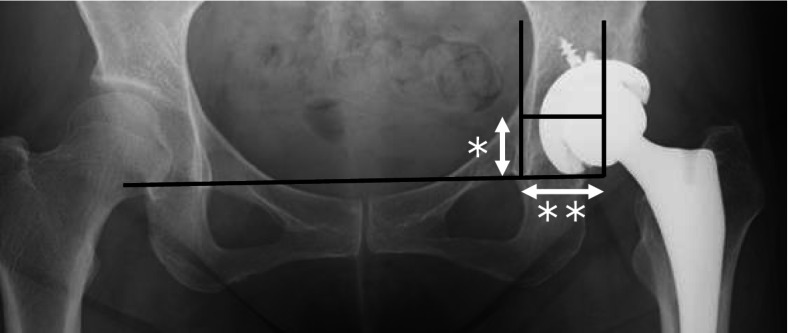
Postoperative anteroposterior X-ray radiogram of THA. The vertical distance (*) is defined as the distance from the lower edge of the bilateral the tear drops to the center of the hip joint. The horizontal distance () is defined as the distance of the horizontal direction from the lower edge of the tear drop to the hip joint center. THA: total hip arthroplasty
